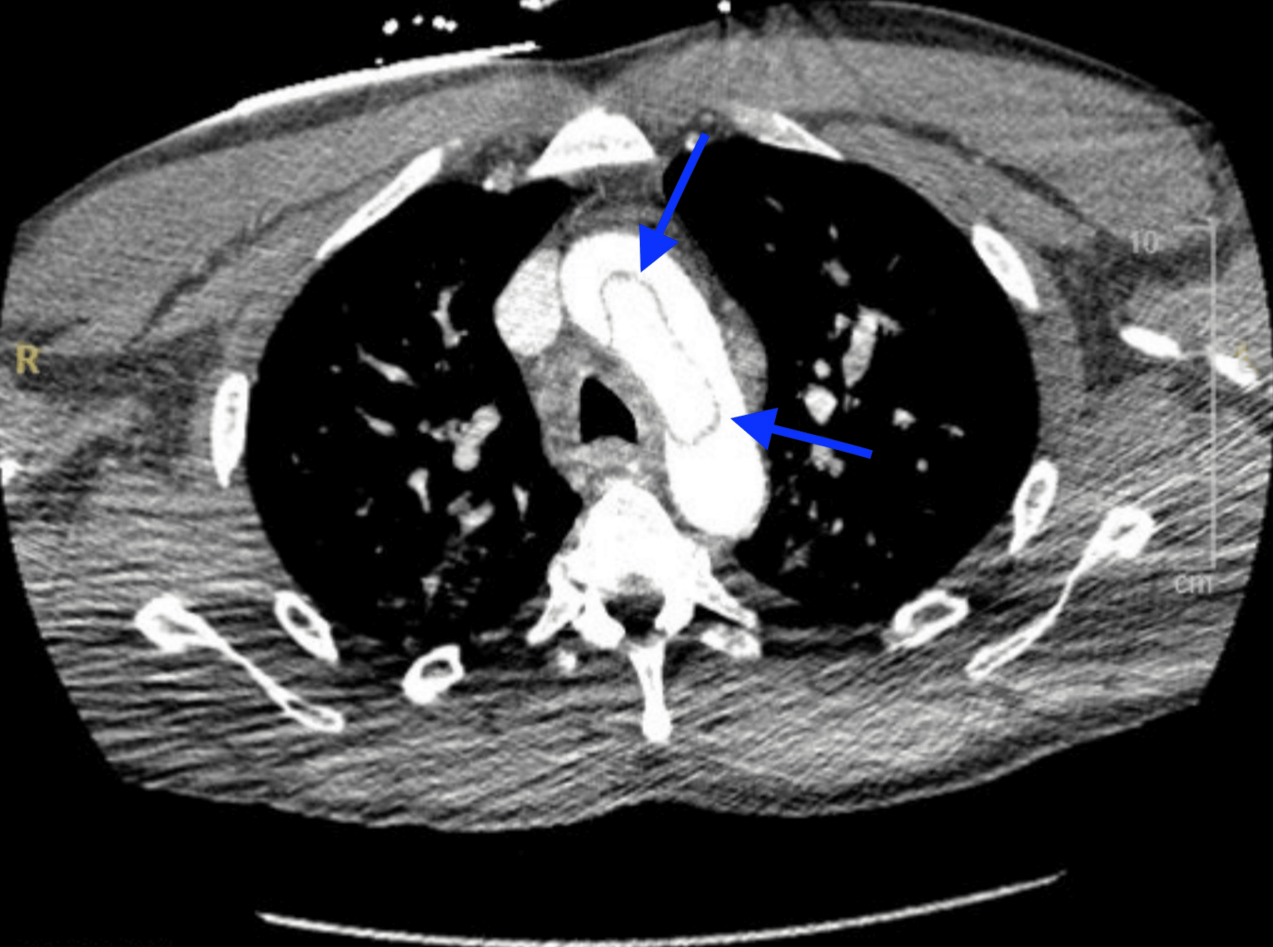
Transverse section of CT angiography of the chest showing an aortic dissection involving the aortic arch, as indicated by the blue arrows.
